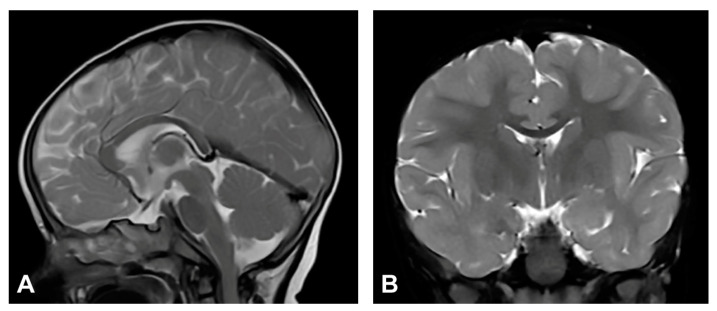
(Case 4) (A) Sagittal and (B) coronal T2W magnetic resonance images at the age of nine months without pathological findings.
